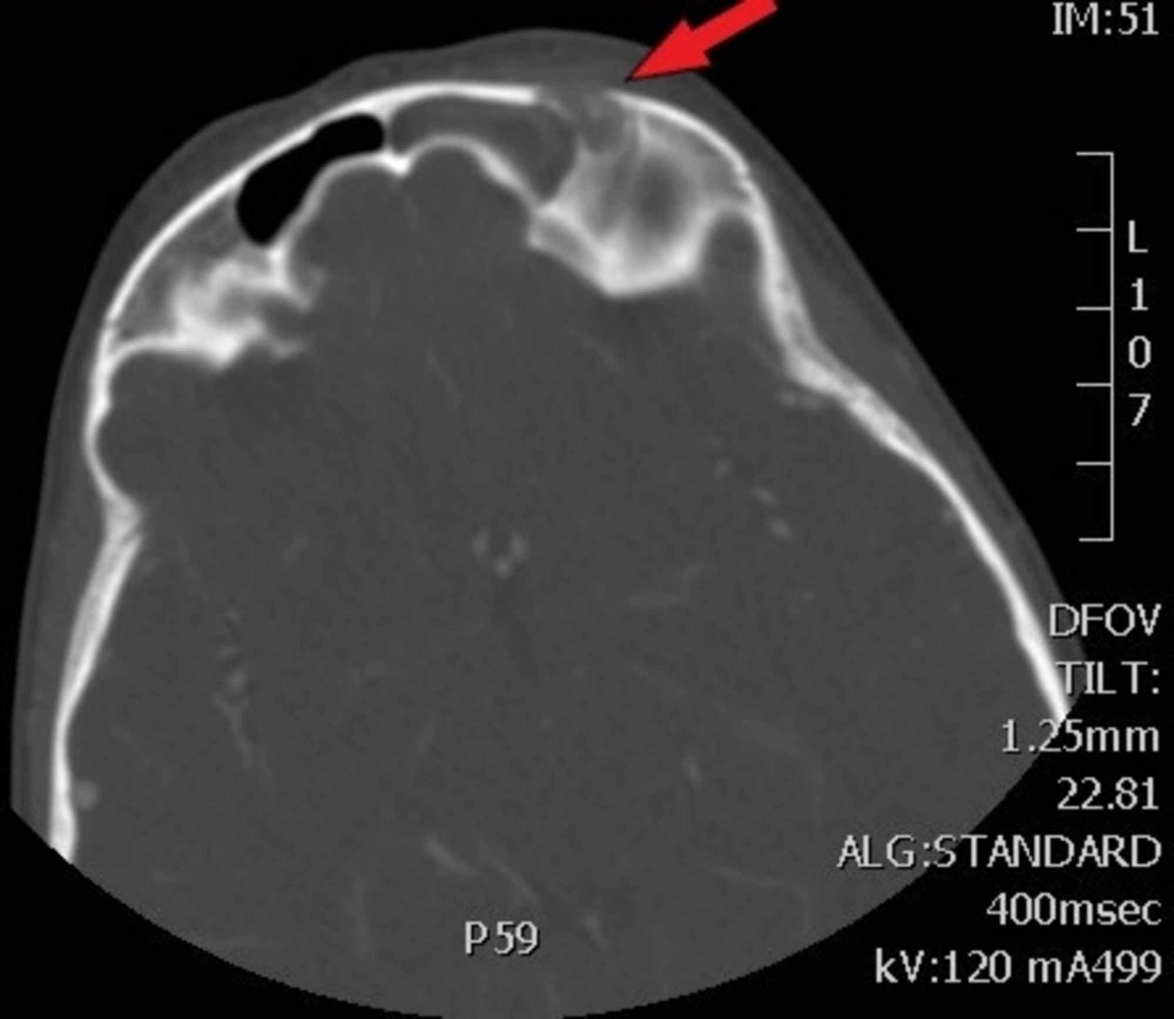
Axial CT scan with arrow indicating erosion of the left frontal bone and opacification of the left frontal sinus
